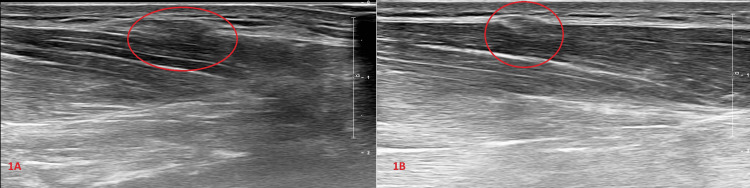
Dynamic ultrasonography of bilateral tibialis anterior muscle herniation A (right), B (left): Longitudinal ultrasonographic images showing focal fascial defects at the middle third of both crura, with protrusion of the tibialis anterior muscle through the fascia. The red circles indicate the herniation sites.
